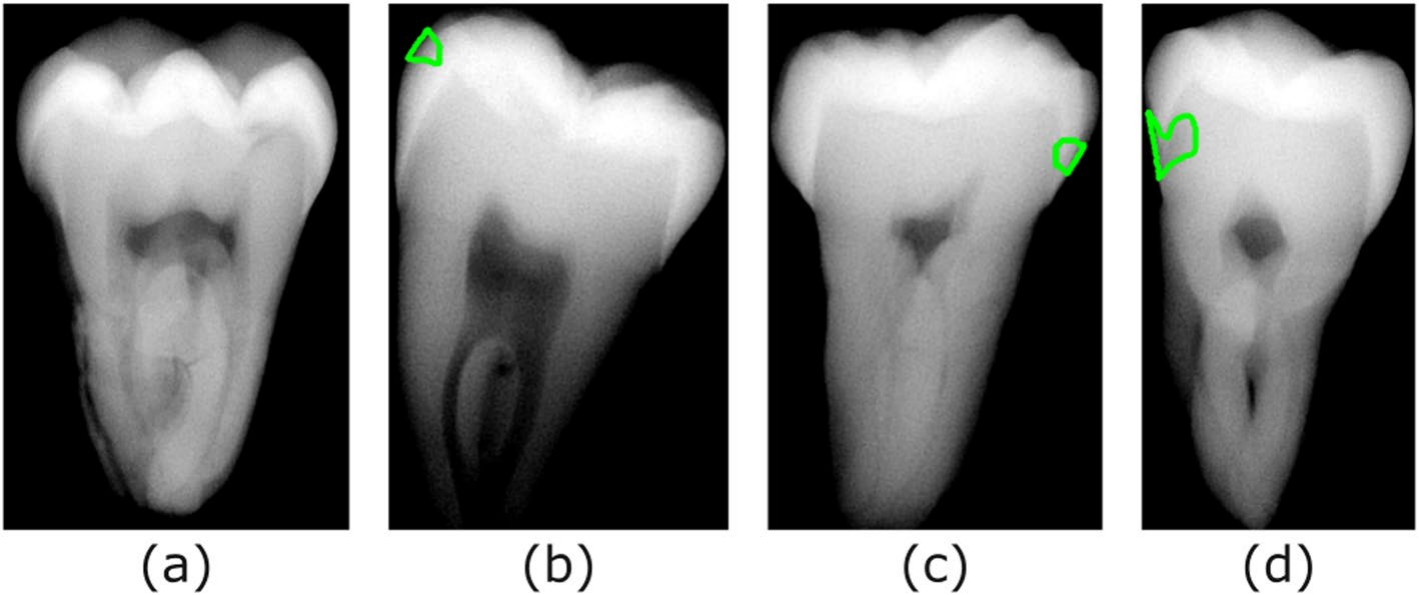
In-vitro radiographs showing caries lesion annotations. The corresponding perceived caries lesion stages are: a Sound, b RA1, c RA2, d RA3
